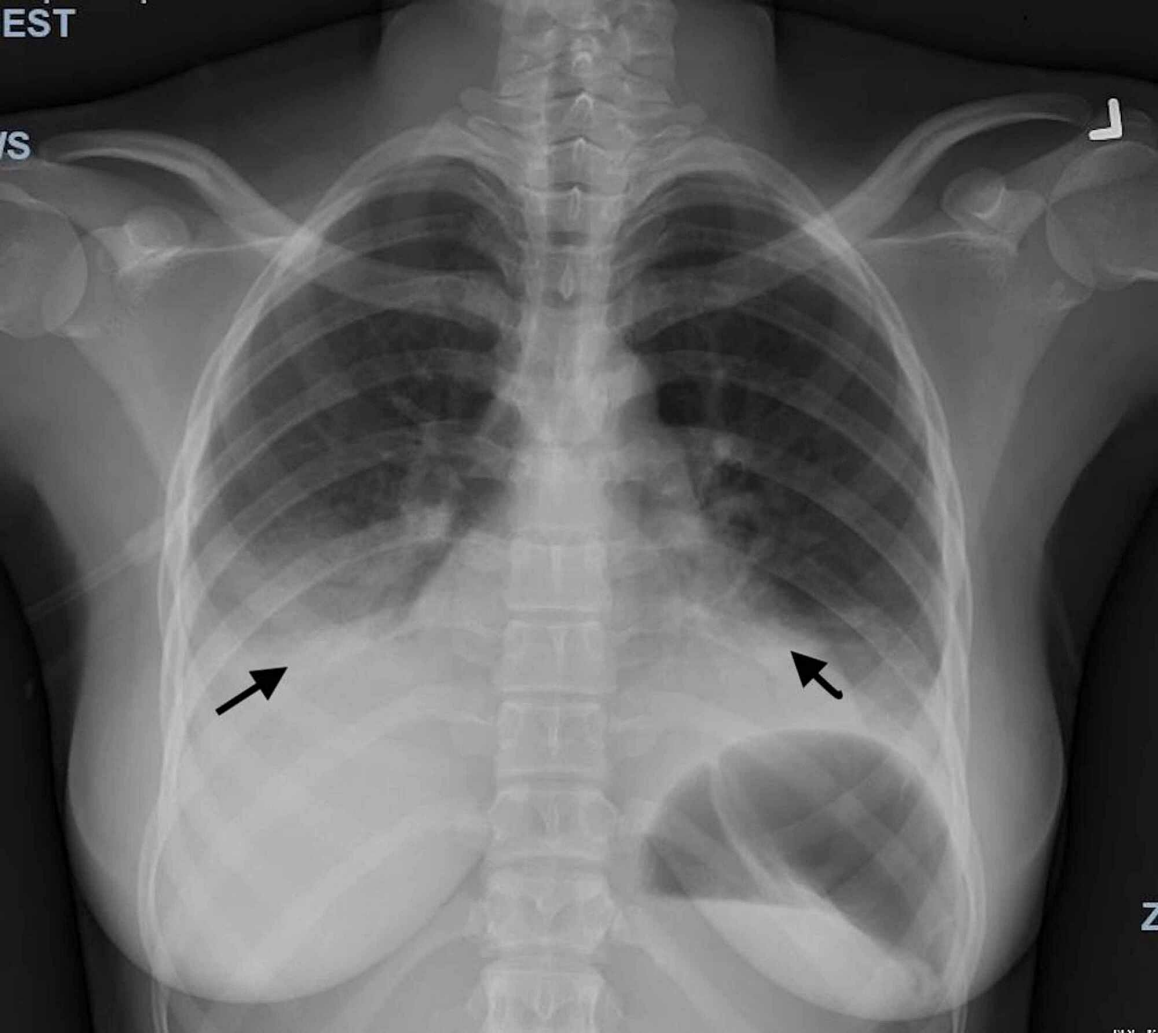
Chest X-ray (PA and lateral) The image shows a further increase in the ground-glass opacities within both lungs with increased opacity within the retrocardiac region with air bronchogram. Worsening airspace disease indicative of pneumonia is also observed PA: posteroanterior
